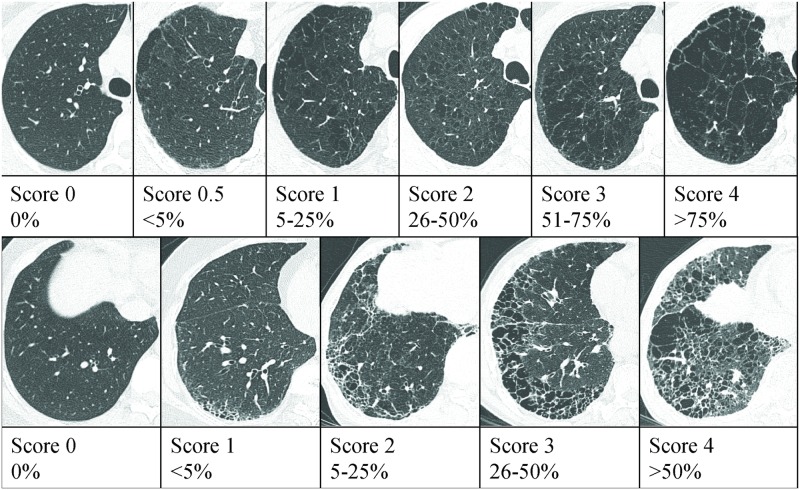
CT images for standard reference cases of emphysema and fibrosis. (A) The extent of emphysema was visually scored on a six-point scale. (B) The extent of fibrosis was also visually assessed on a five-point scale for reticulation, honeycombing, and total fibrosis.
